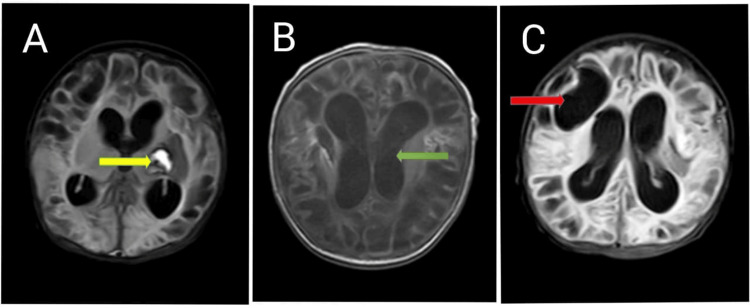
Pre discharge MRI revealed. (A) Hemorrhage in the lentiform nucleus (yellow arrow), (B) Dilatation of bilateral lateral ventricles (green arrow), (C) Cystic encephalomalacia (red arrow).
